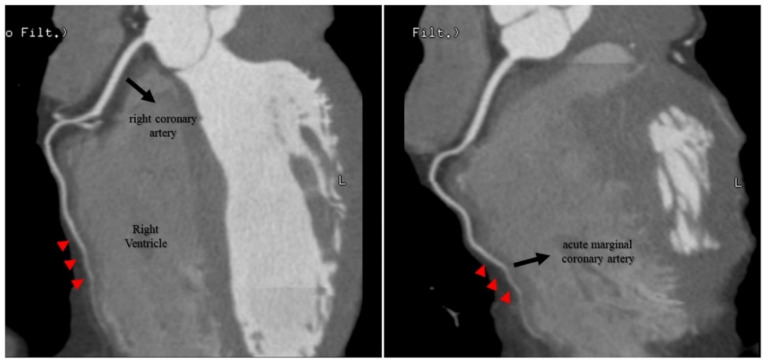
RAM intramyocardial segment shown in CCTA imaging. CCTA demonstrated that the RAM was partially surrounded by myocardium (red arrowheads), being adjacent to the right ventricular cavity in a short extension of its course along the right ventricular free wall. CCTA: coronary computed tomography angiography; RAM: right acute marginal coronary artery.
